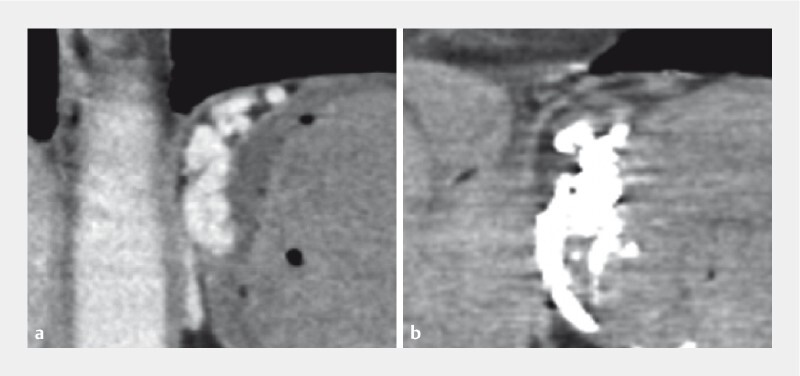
Computed tomography scan evaluation after cyanoacrylate injection. The scan showed good pooling of cyanoacrylate with lipiodol after injection.
